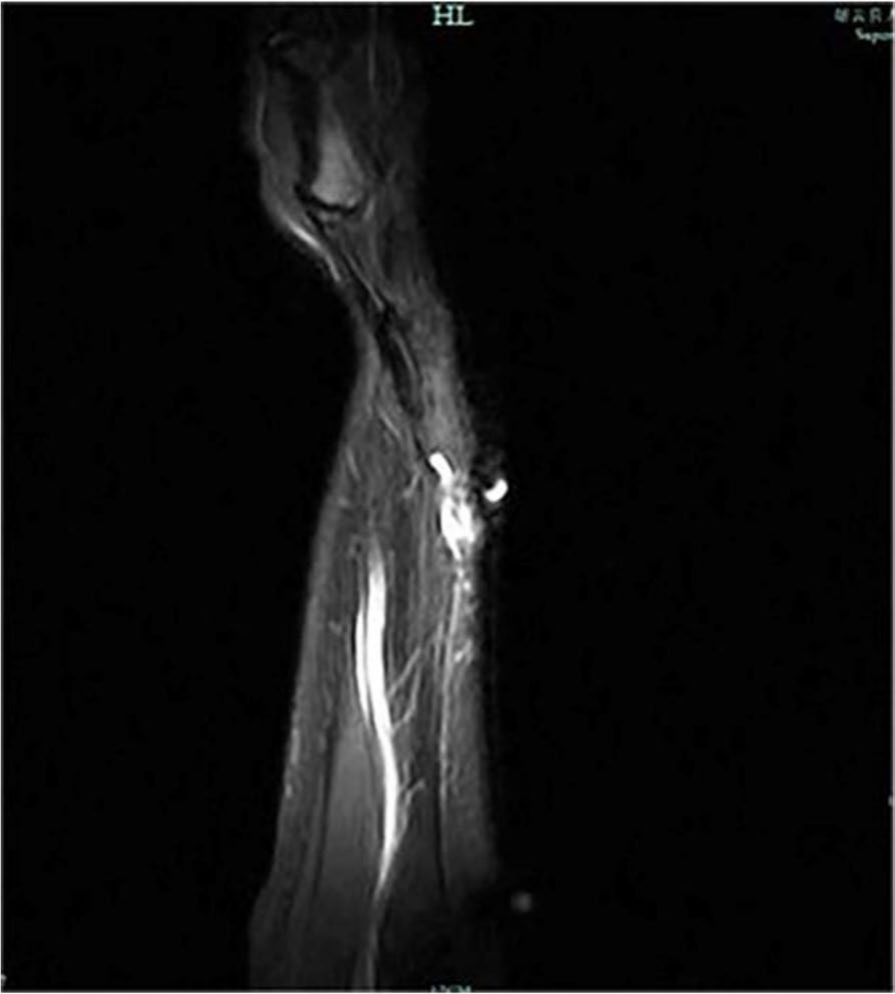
MRI shows inflammatory high signal
